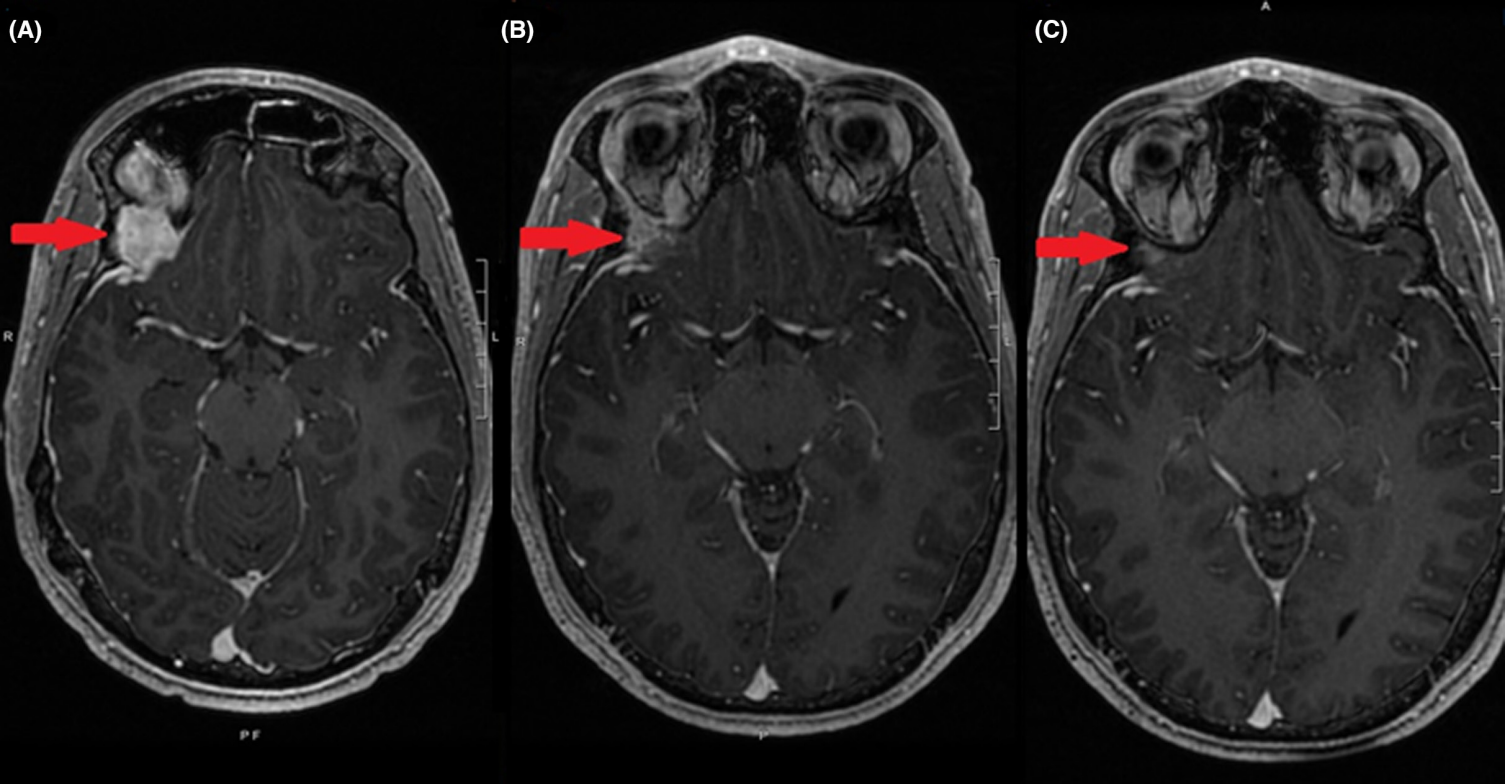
Regression of the right orbital Langerhans cell histiocytosis. Red arrow points to the initial site of disease. (A) MRI brain axial T1 post contrast 2 months after partial surgical resection (B) MRI brain axial T1 post contrast 3 months from radiation (C) Most recent MRI brain axial T1 post contrast, 1.5 years from the radiation.
